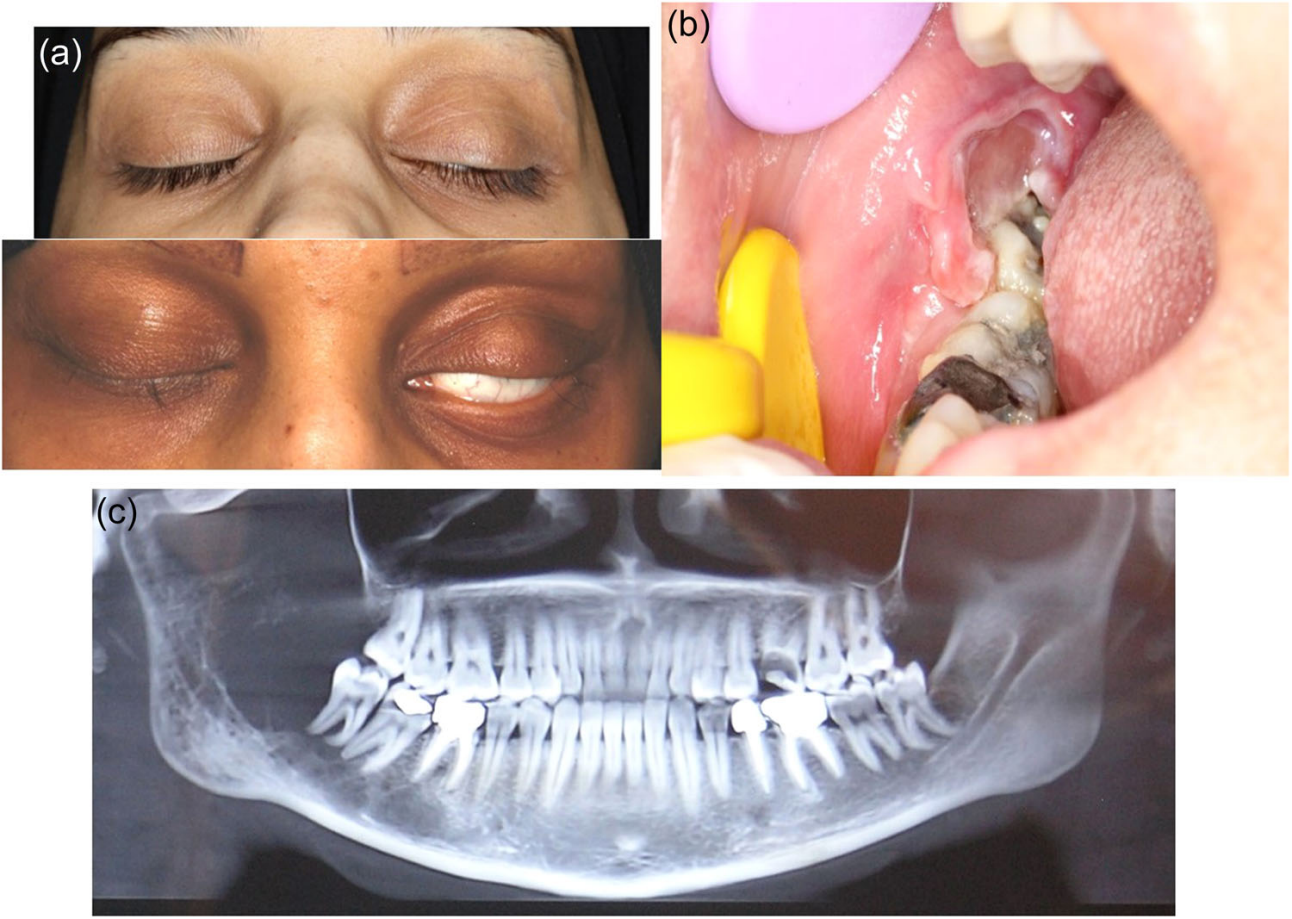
Metastatic carcinoma in a young woman without a history of cancer. She had swelling of the right cheek and toothache (right lower third molar) for a month and right chin's skin paresthesia for eight months before her first visit. She lost about 30 kg of weight within 10 months. She was also suffering from chest, leg, and back pain and (a) inability to close the left eyelid. Antibiotic and NSAIDs had been prescribed by a dentist and a physician before she was referred to a dental school. Breast cancer was discovered after necessary analyses as the primary cancer and widespread metastases were found. (b) Intraoral view. (c) Presence of radiolucency in the involved area in the panoramic radiograph.
